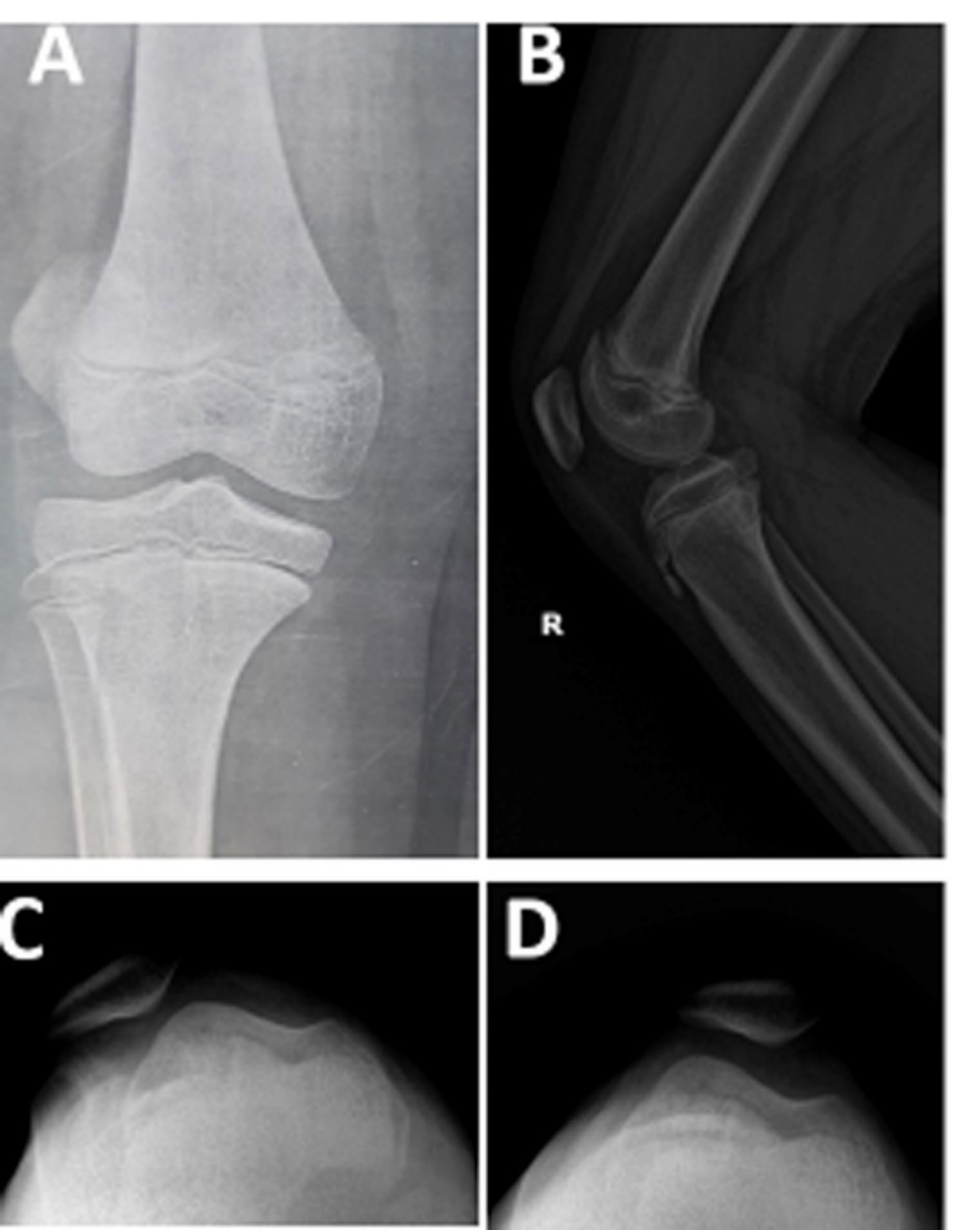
Preoperative and postoperative radiography. (a) True antero-posterior view. (b) True lateral view of the right knee. (c) Axial view of the right knee in 40^0^ knee flexion, showing a laterally dislocated patella. (d) Postoperative axial view of the same knee in 40^0^ knee flexion, showing a well-reduced patella
